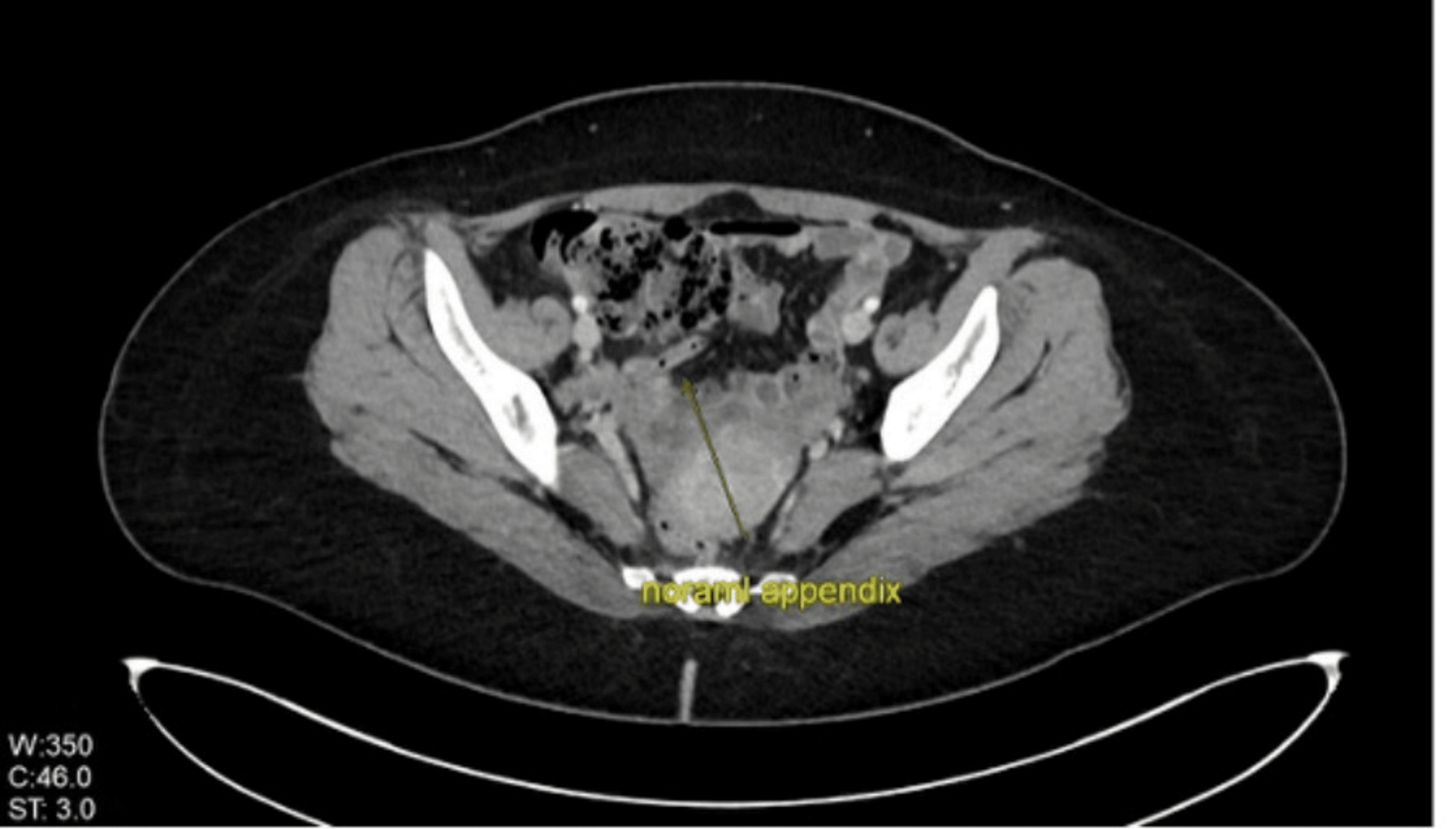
Computed tomography imaging of the abdomen. Computed tomography imaging of the abdomen during the follow-up of the patient, which showed a normal appendix with no signs of inflammation.
